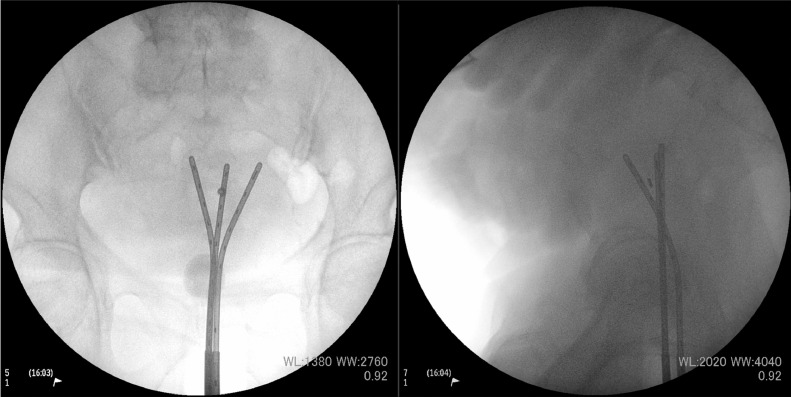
Anteroposterior and lateral fluoroscopic x-ray images of the combination of the Rotte Y applicator and a Fletcher intrauterine 30° tandem during brachytherapy.
